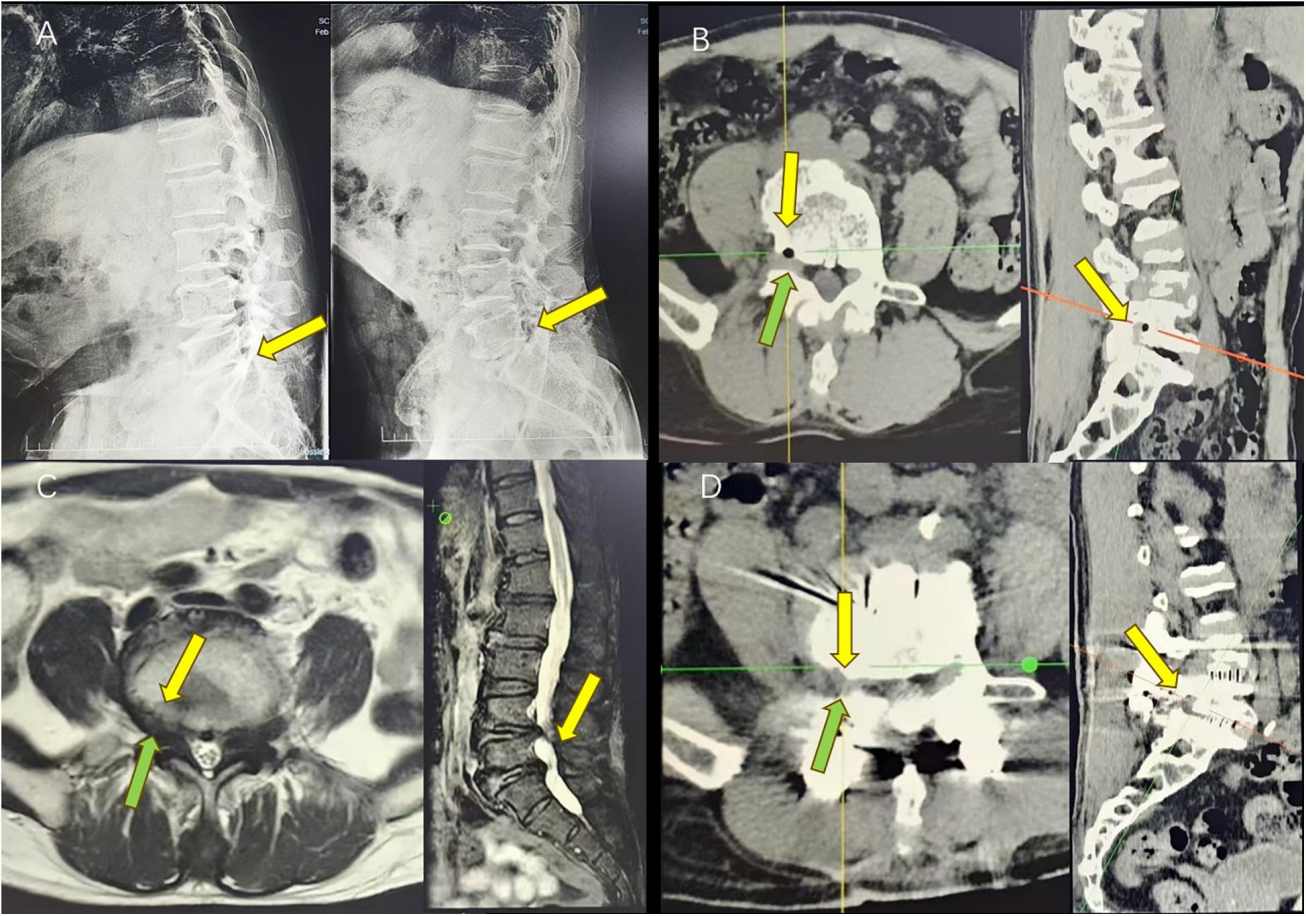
(A) Preoperative DR shows a reduction of the intervertebral heights of L4/5 and L5/S1 and I° slip of the L5/S1 vertebral body (yellow arrow). (B) Preoperative CT suggests that the L5/S1 disc is vacuumed, and the right disc is herniated with a vacuole (the yellow arrow indicates the location of the herniated disc) and the corresponding nerve root is compressed (the green arrow indicates the location of the compressed nerve root emanation). (C) Preoperative MRI shows T1/T2 hypodensities (the yellow arrow indicates the location of the herniated disc), with the corresponding nerve root compression (the green arrow indicates the location of the compressed nerve root emanation). (D) Postoperative CT shows the disappearance of the cyst (the yellow arrow indicates the location of the original cyst), with nerve root relaxation (the green arrow shows nerve root relaxation).
